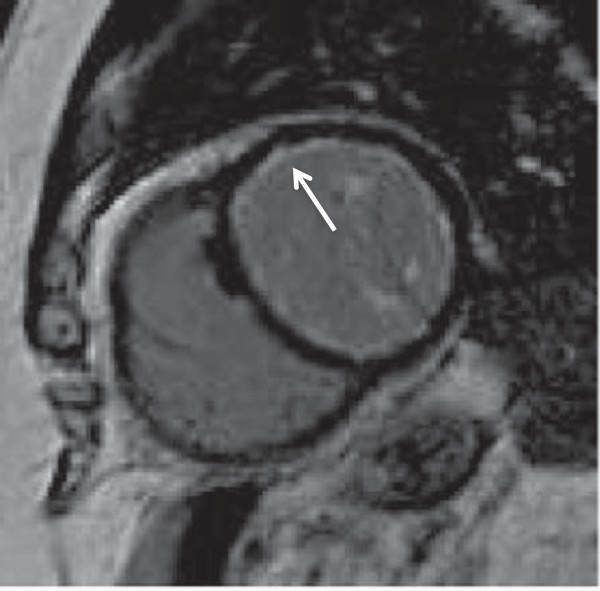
Magnetic resonance imaging with arrow depicting circumferential subendocardial delayed enhancement on the short axis view.
